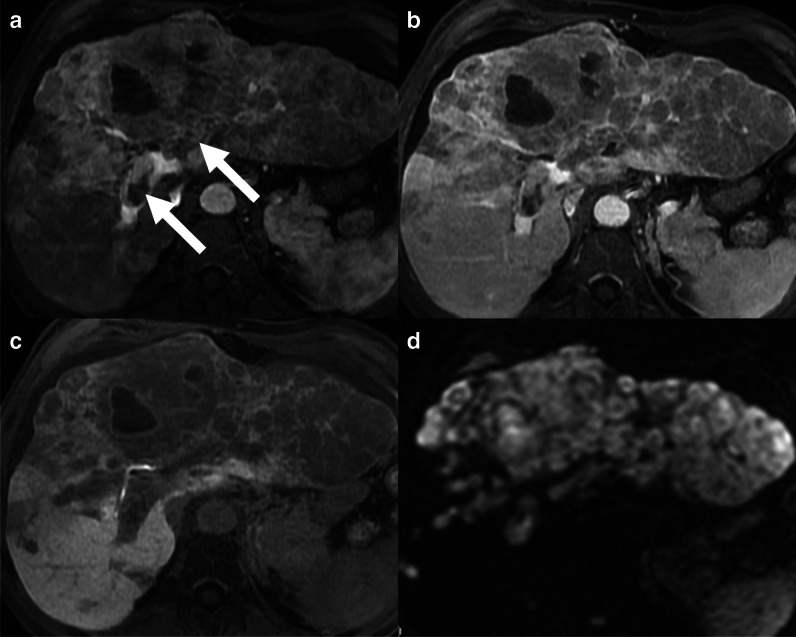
A 58-year-old man with cirrhosis and infiltrative hepatocellular carcinoma with macrovascular invasion. MRI acquired after the administration of gadoxetate disodium shows a bilobar infiltrative lesion with non-rim arterial phase hyperenhancement and macrovascular invasion in the left and right portal vein branches (a, arrows), non-peripheral washout on portal venous (b), hypointensity on the 20-min hepatobiliary phase (c), and marked restricted diffusion (d)
